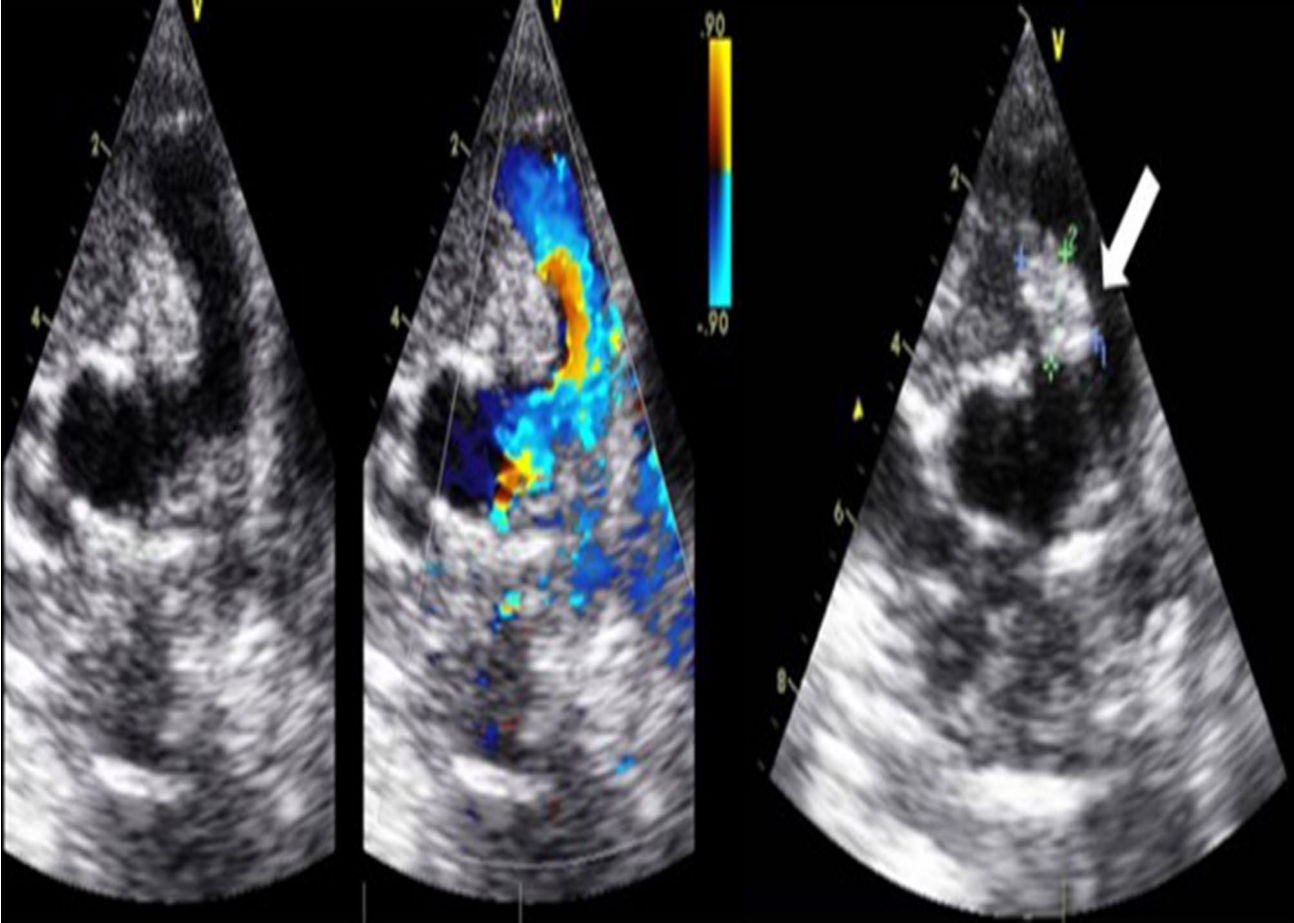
Transthoracic echocardiography showing a single large oval (11 × 13 mm) mass attached to the pulmonary valve (white arrow), it is highly mobile, homogenous, echo dense with smooth surface, no evidence of mural or intramyocardial extension. The mass causes a severe degree of pulmonary incompetence (G III/ IV) while not affecting forward pulmonary flow
